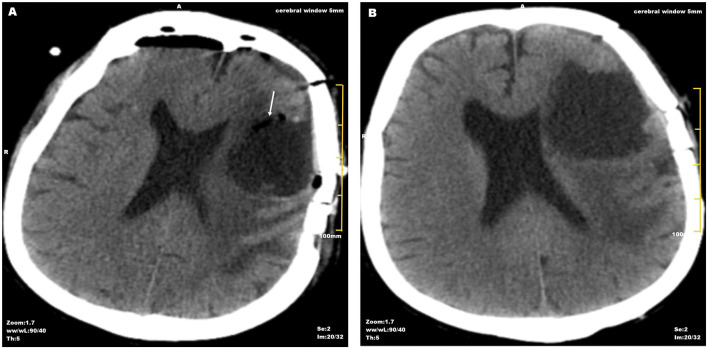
Postoperative computed tomography evolution following neurosurgical drainage. (A) Non-contrast CT on Day 15 showing surgical cavity with residual pneumocephalus (arrows) and mild effusion; midline structures centered. (B) CT on Day 25 demonstrating resolution of pneumocephalus and reduction in lesion size with decreased edema, indicating favorable response to therapy.
